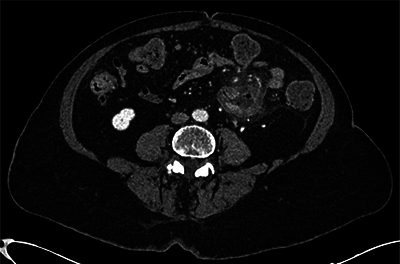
Axial contrast-enhanced CT image revealing jejunal diverticulitis without complications.
